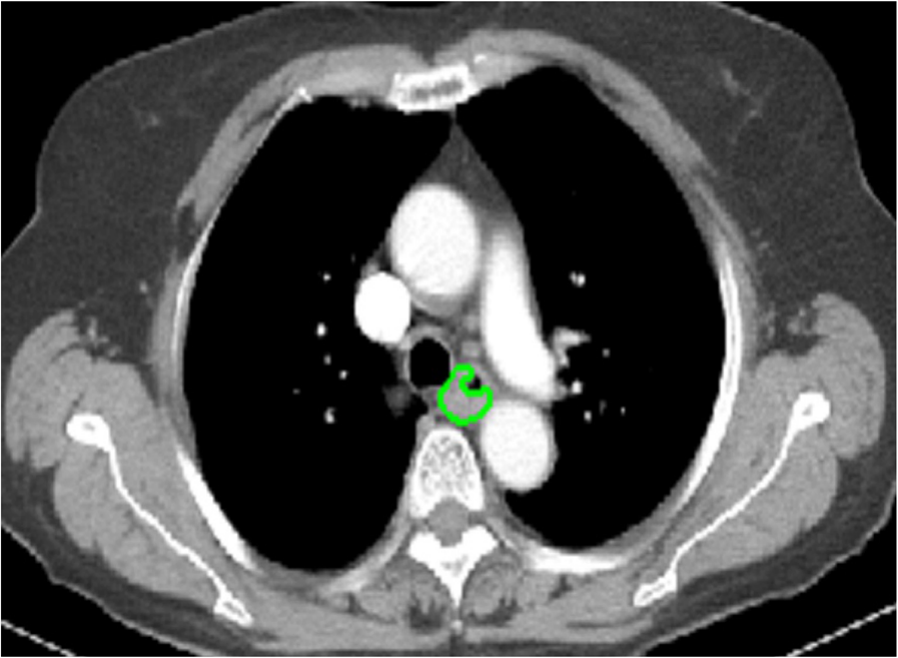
The tumour contours are segmented manually on thoracic contrast-enhanced CT image
